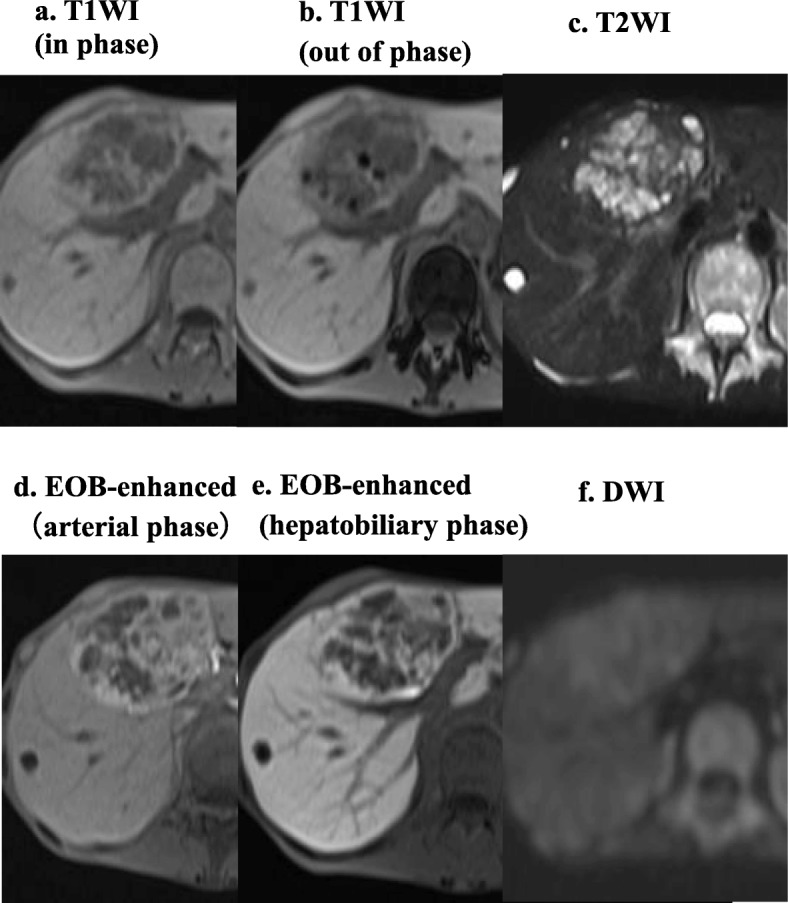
Magnetic resonance imaging. a T1-weighted image (in phase) showing isodense signal intensity. b T1-weighted image (out of phase) showing low signal intensity. The tumor inside had fatty deposition. c T2-weighted image. The central part of the tumor showed high density, and it was accompanied by a cystic lesion. d Gadolinium ethoxybenzyl diethylenetriamine pentaacetic acid (Gd-EOB-DTPA)-enhanced image (artery phase). The tumor was enhanced similar to the results of computed tomography imaging. e Gd-EOB-DTPA-enhanced image (hepatobiliary phase). The enhancement remained. f Diffusion-weighted images showed slightly high density
